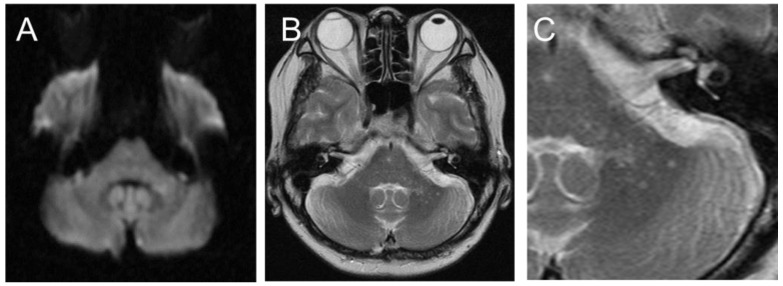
Initial brain magnetic resonance imaging (MRI) showed a small, high-intensity lesion in the left cerebellar peduncle on diffusion-weighted imaging (A). On T2-weighted imaging (B), the lesion appeared to be a cluster of small spots ((C): enlarged image of (B)).
